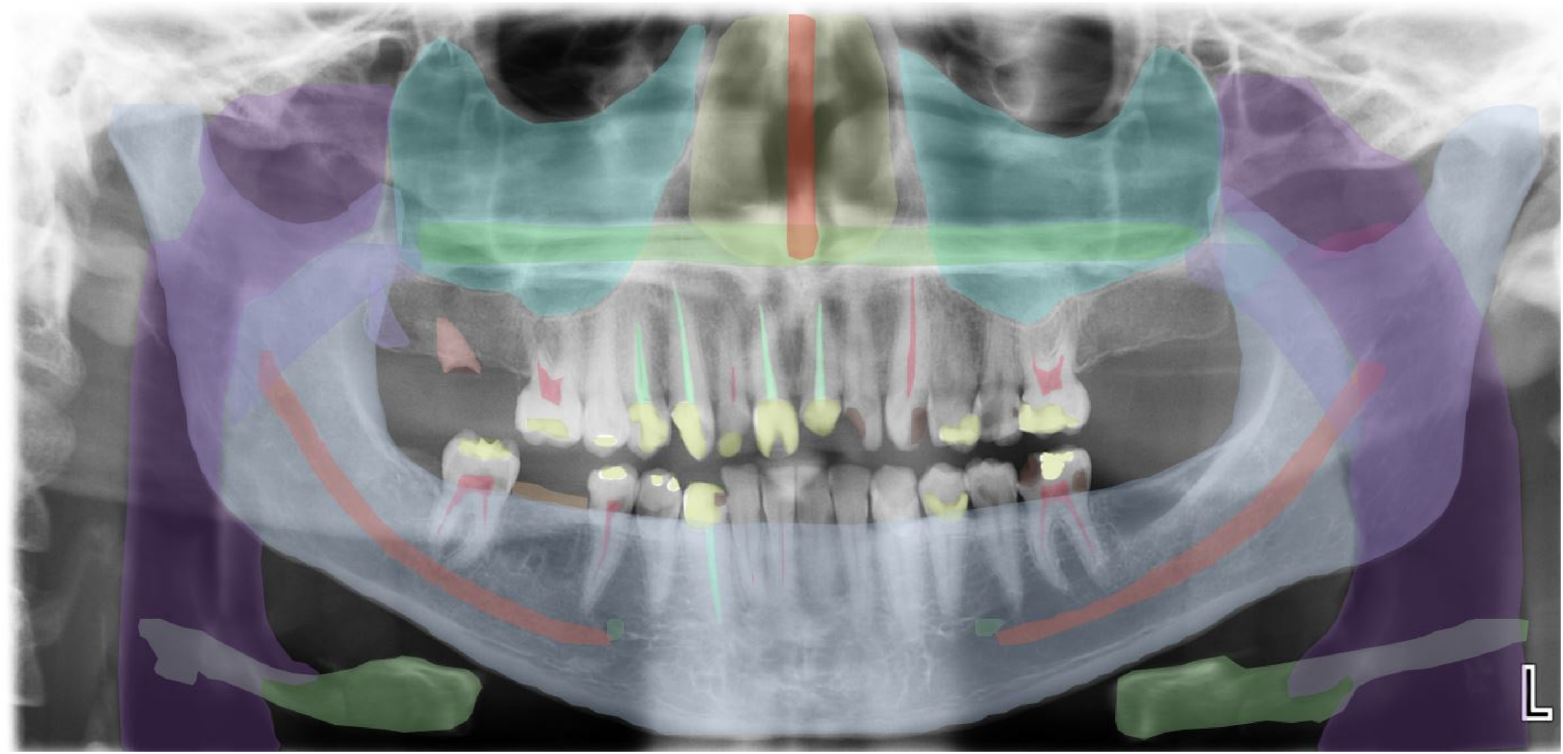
Automatic segmentation of a panoramic radiograph showing normal anatomy of oral and maxillofacial area.
